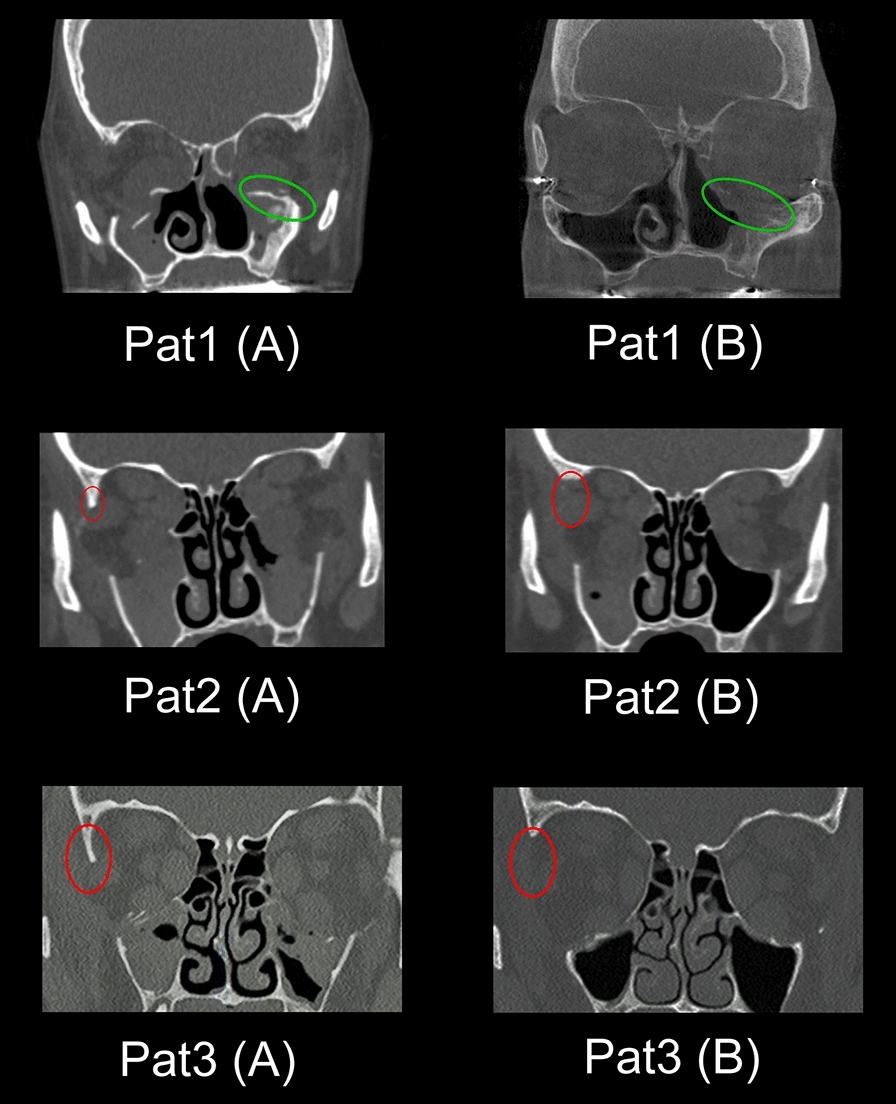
Post-surgery cross-section images. Cross-section images of post-orbital decompression (left A column) and re-decompression (right B column) CT data for three patients (no. 1, 2, 3 in Table 1. Green ellipses indicate remaining A re. resected B key point 2 in CT cross-section images of the patient 1. Red ellipses indicated remaining A re. resected B key point 2 in CT cross-section images of patients 2 and 3
